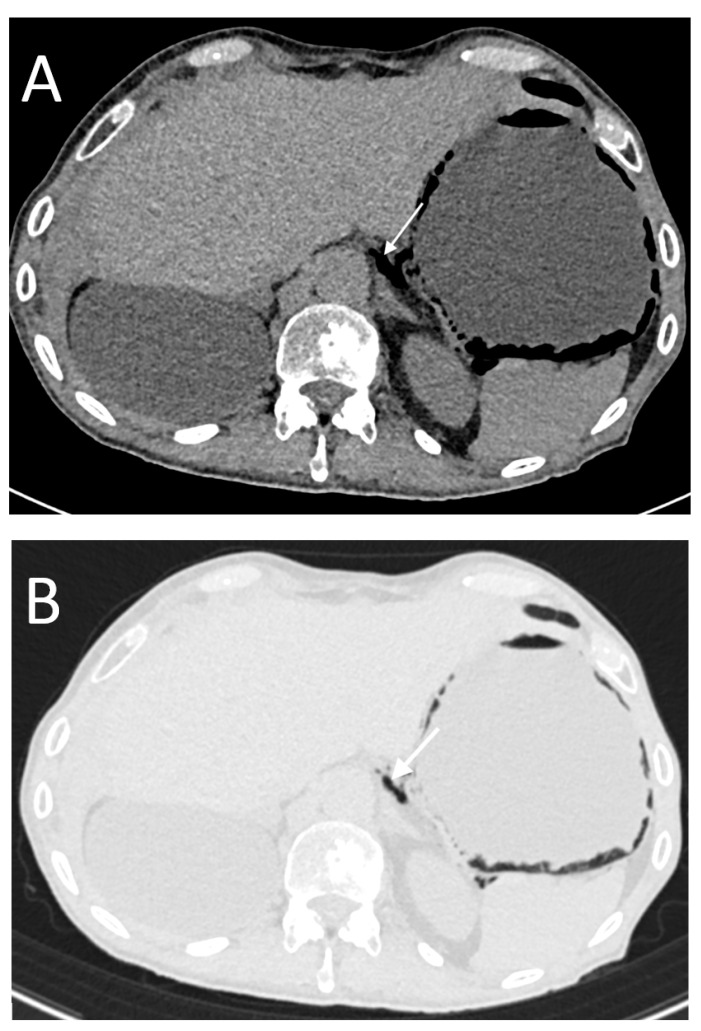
A 61-year-old male with perforation of the posterior wall of the stomach. The precontrast CT scan shows retroperitoneal free air (arrow in (A,B)) only, with no evidence of gastric wall breach. Note the diffuse parietal pneumatosis of the gastric wall.
